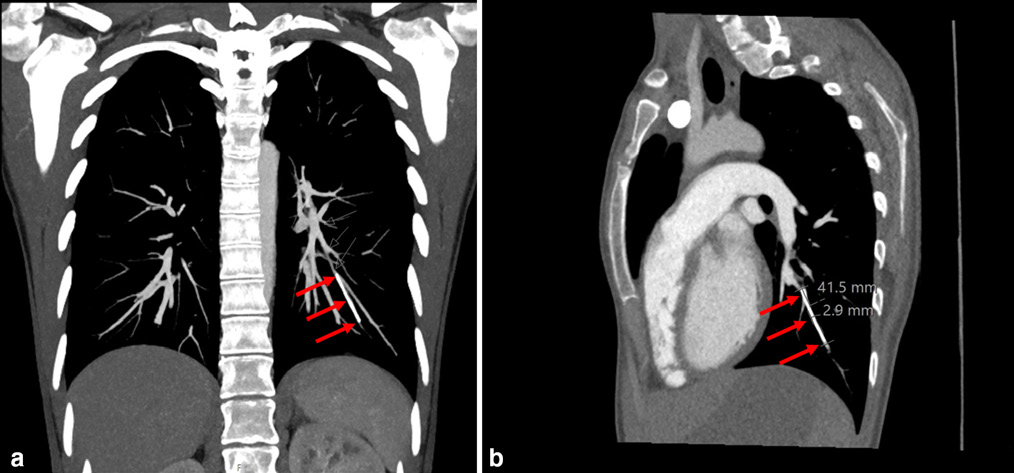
(a) Coronal section of CT pulmonary angiogram with arrows demonstrating 4 cm linear opacity in subsegmental branch of the pulmonary artery supplying the left lower lobe. (b) – Saggital section of CT pulmonary angiogram with arrows demonstrating 4 cm linear opacity in subsegmental branch of the pulmonary artery supplying the left lower lobe
